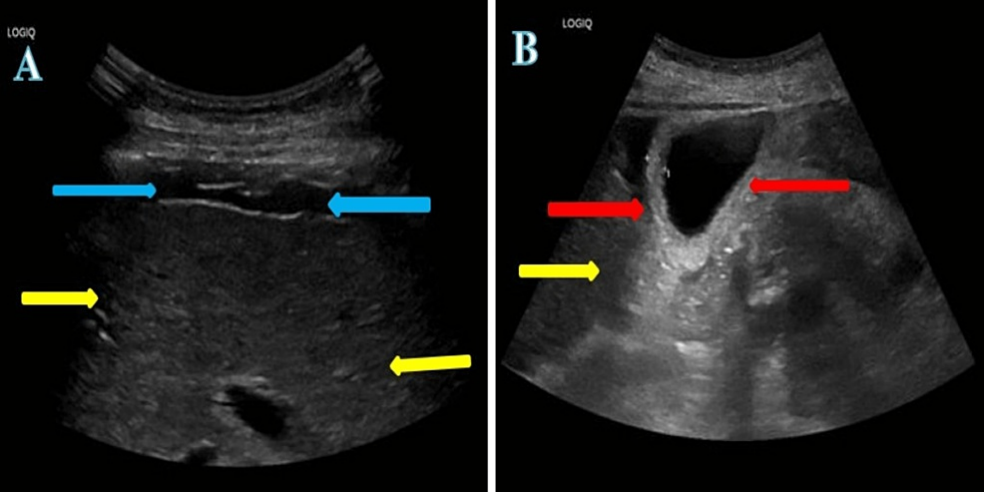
Ultrasound images A and B: ultrasound liver demonstrates mild irregular margin with coarse texture (yellow arrows) and markedly thickened gallbladder wall (red arrows), with mild to moderate ascites (blue arrows).
